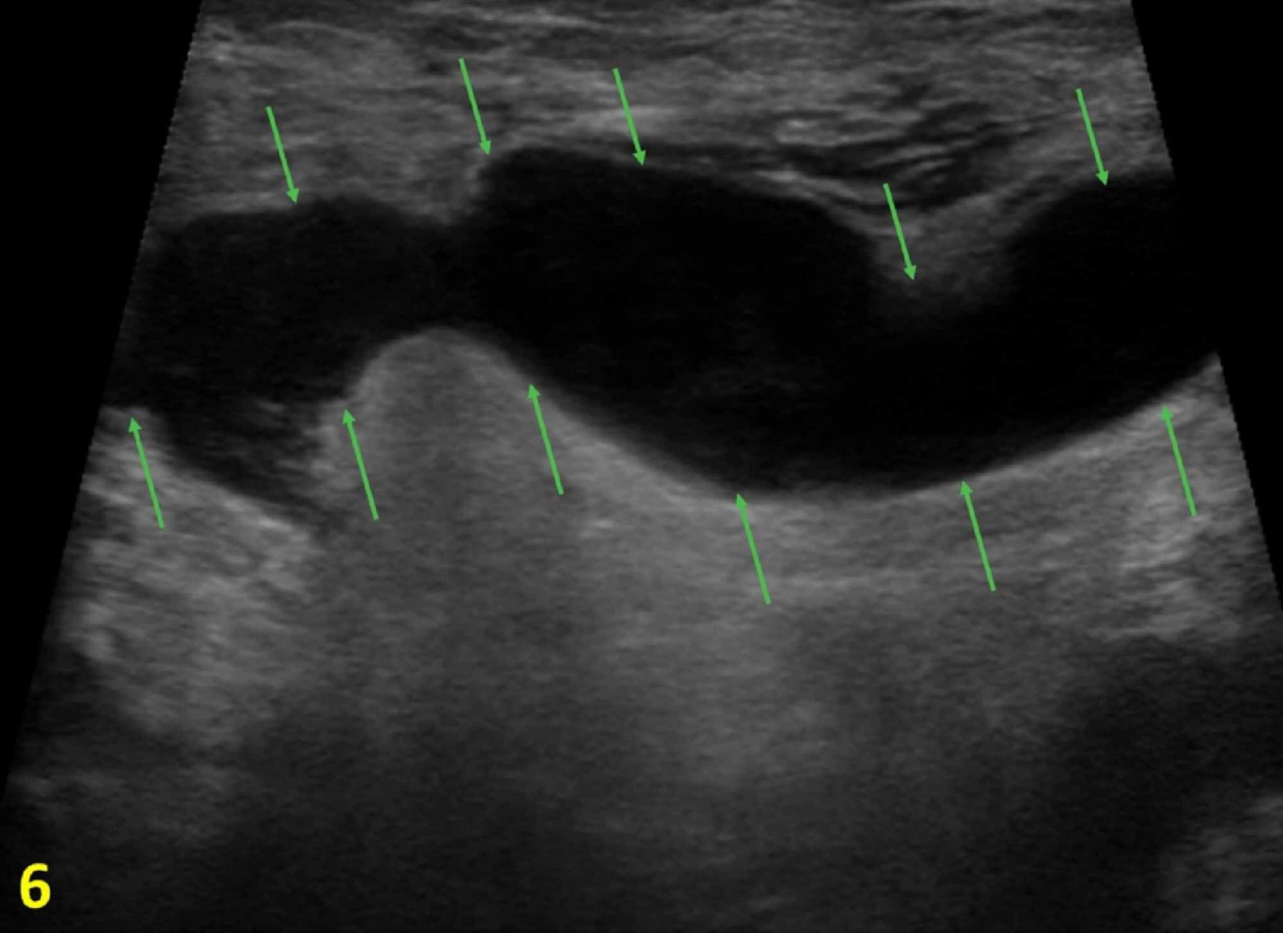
Right Axilla Diagnostic Ultrasound The image shows axillary lymphadenopathy with cortical thickening measuring 31 mm (outlined by green arrows).
